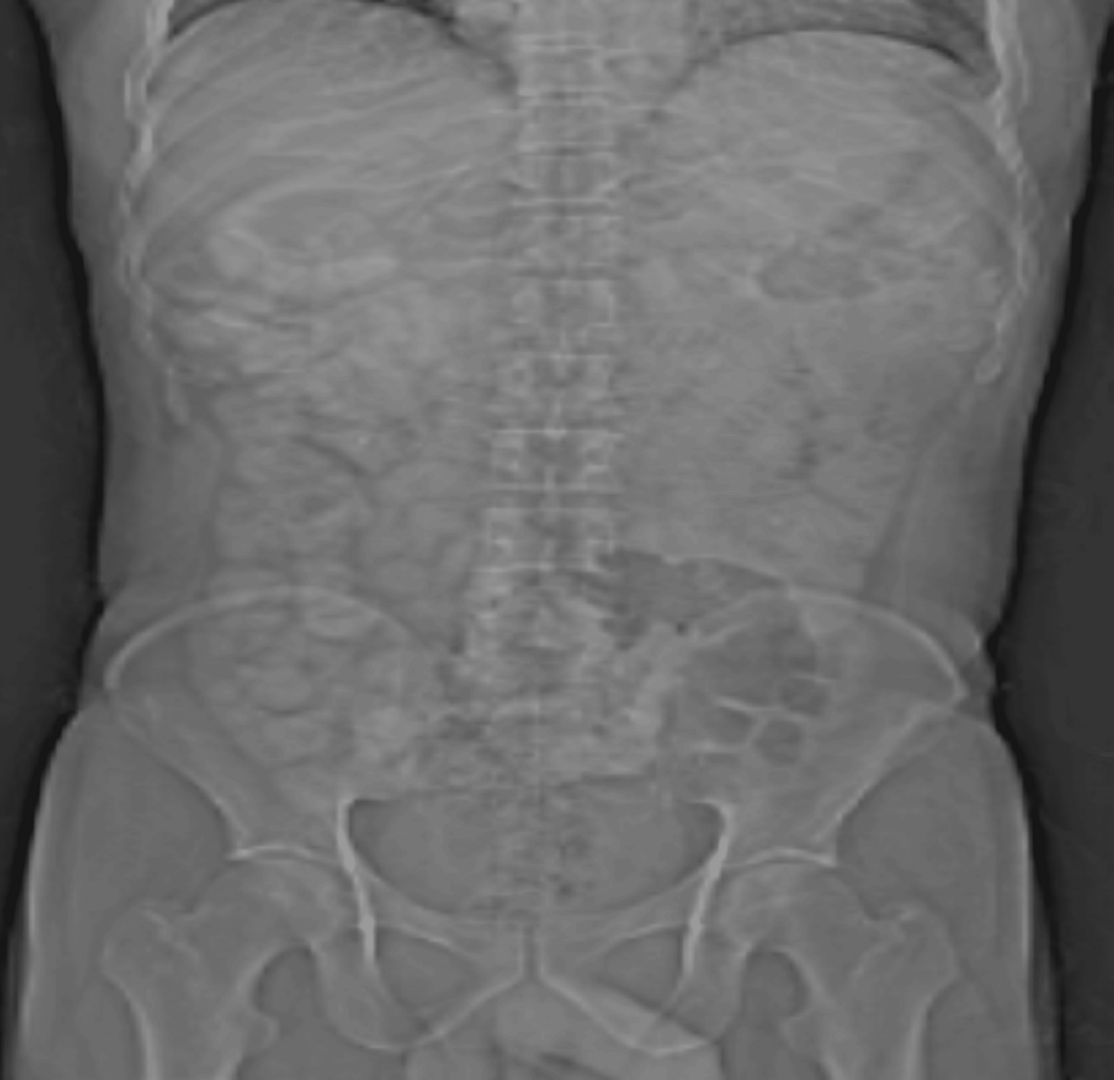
Abdomen radiograph showing bowel distention with mild ascites
